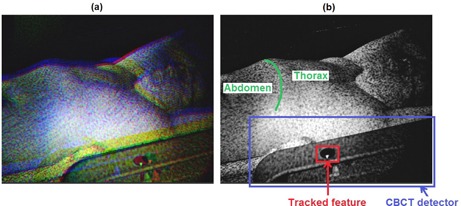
Video image (a) acquired by the right‐side imaging pod of the AlignRT system. The images captured by the three cameras of the pod are superimposed as RGB components. The slight offset between the three components is due to the different position of the cameras in the imaging pod. Blue image component (b), depicting the CBCT flat‐panel detector and the elliptic feature used to track the detector motion. The abdominal and thoracic regions of patient surface are also represented, highlighting the costal margin as separation line.
